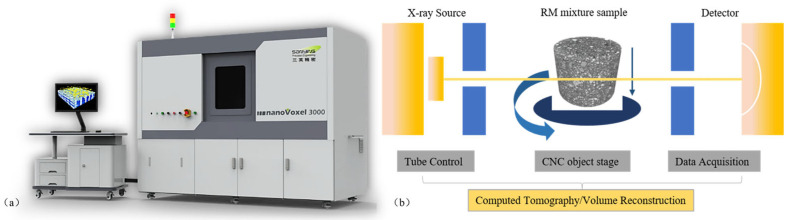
(a) CT scanner; (b) working principle.
